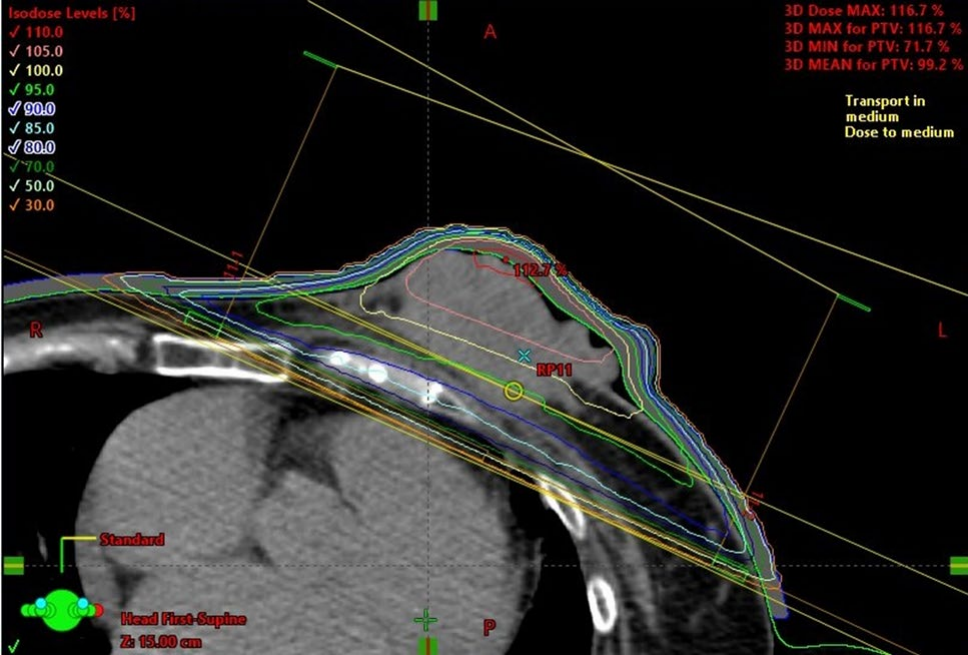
Representative axial CT slice from the first treatment, showing the distribution of the radiation isodose line
